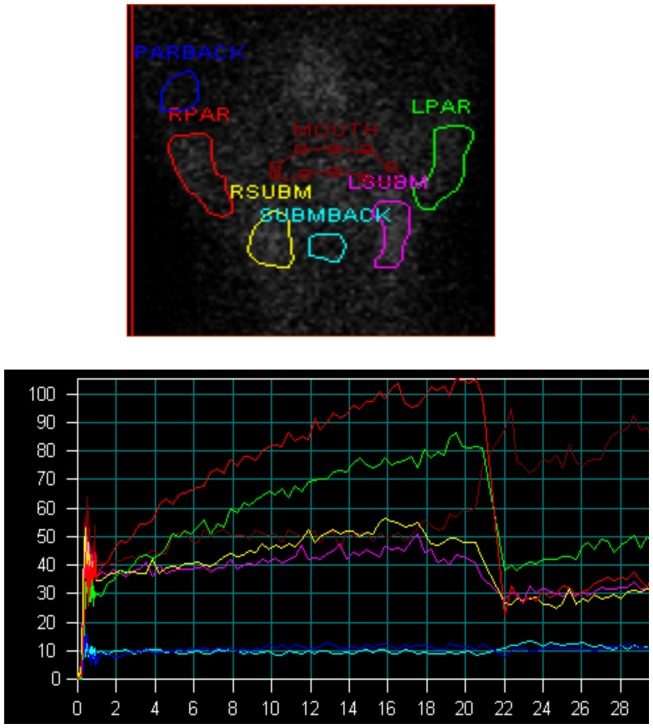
Salivary scintigraphy. Upper figure shows regions of interest, lower figure shows time activity curves (*y* axis = counts per second, *x* axis = minutes, lemon juice was administered at the 20 min time point). Red = right parotid, green = left parotid, yellow = right submandibular gland, pink = left submandibular gland, blue = parotid background, turquoise = submandibular background, brown = mouth.
